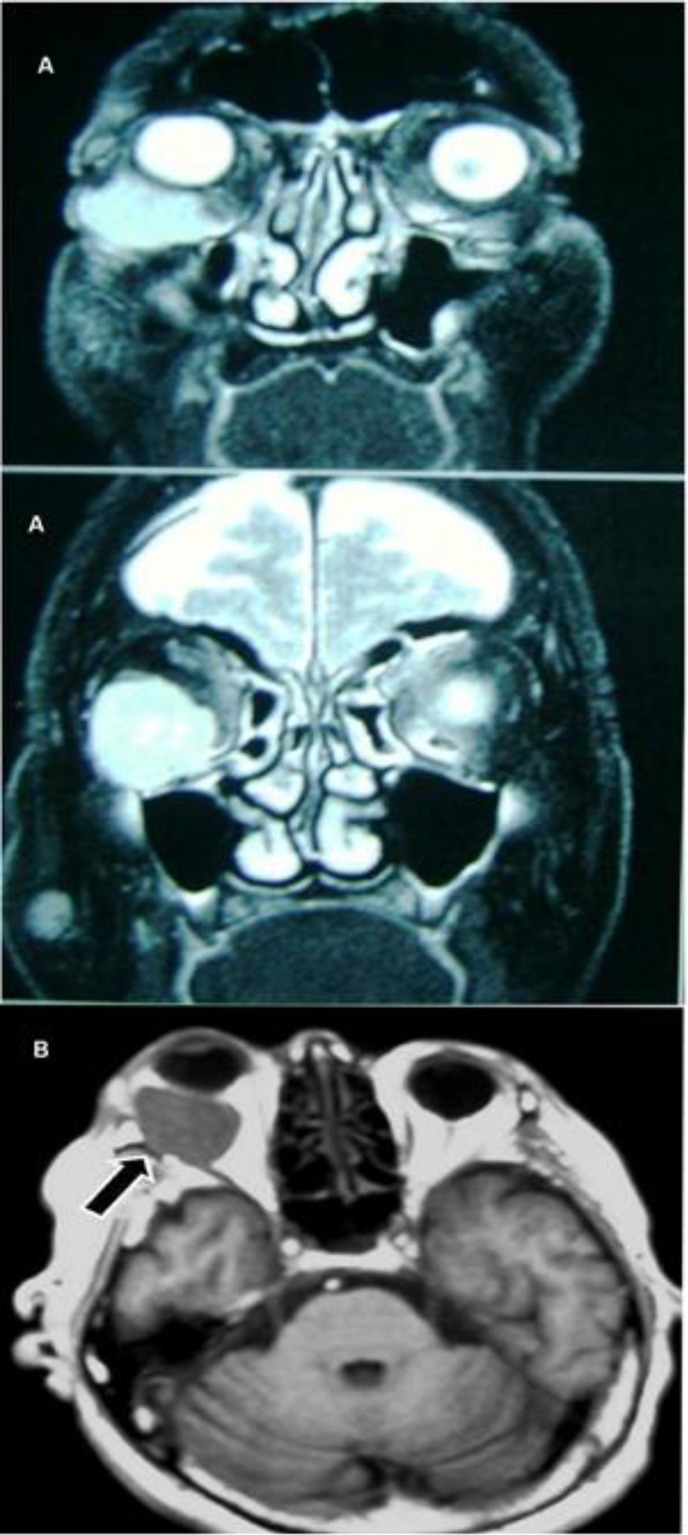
Α) Exophthalmos of the Right Eye, and Β) MRI (Black Arrow Indicates the Lesion).
